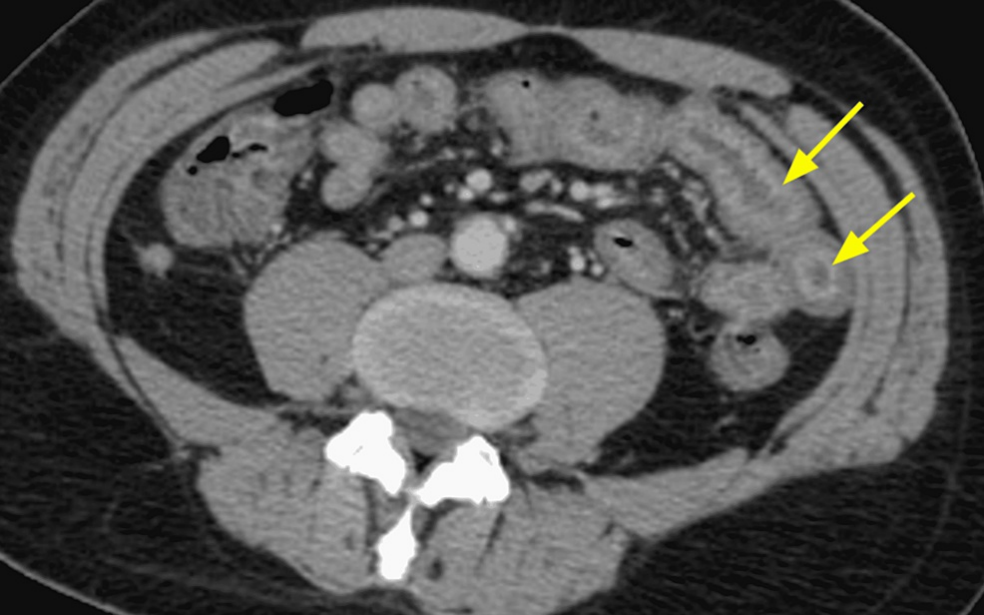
Contrast-enhanced CT image for Case 1 (axial plane) showing thickening of the colonic wall and increased mucosal enhancement (arrows)
